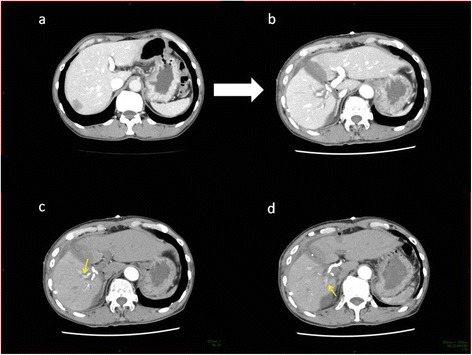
CT scan (a) before and (b) 6 days after the initial ALPPS, showing marked FLR enlargement. (c,d) arterial phase imaging. An arterioportal shunt was formed in the right Glissonian pedicle (yellow arrow).
